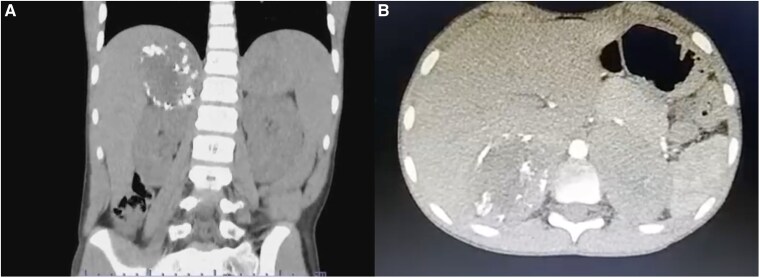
Coronal (A) and axial (B) CT images showing bilateral adrenal masses, with calcification at the periphery of the right adrenal mass.
